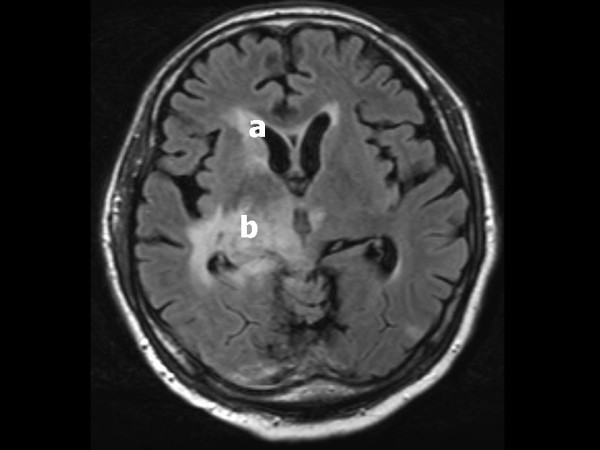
Axial *T*_2_-weighted magnetic resonance imaging of our patient at day 16 after admission shows an abnormal signal at (a) the corpus callosum and right-sided caudate nucleus as well as (b) the right-sided lentiform nucleus and medial geniculate nucleus extending into the right thalamus and temporal lobe. These abnormal signals are consistent with tuberculomas and lepto-meningeal infiltration of the thalamic region and the basal nuclei by *Mycobacterium tuberculosis*.
